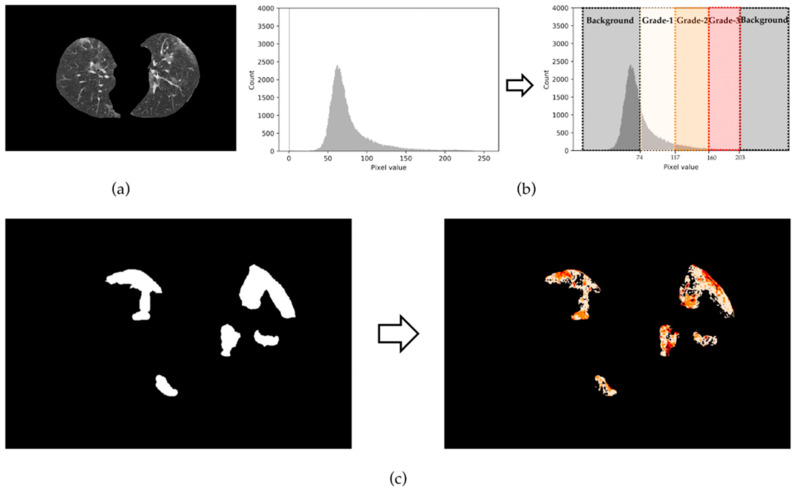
Visualization of label refinement (g = 3): (a) is the lung image segmented from a CT image. The left part of (b) is the histogram of (a) and we grade the pixels based on the pixel distribution of the infected and uninfected areas in the lung; (c) shows the lesion labels before and after the refinement.
